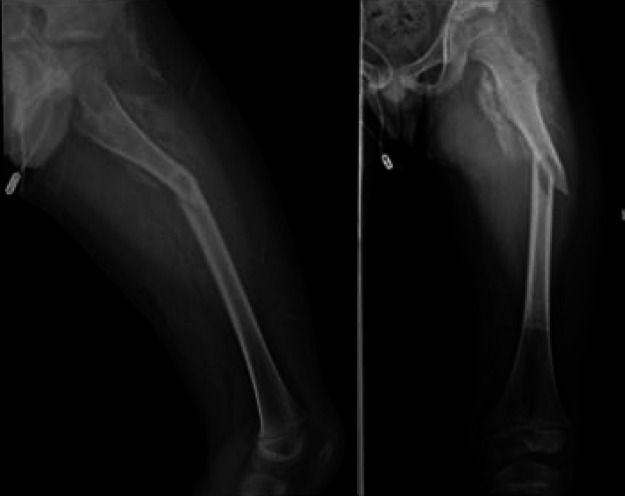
The x-ray of community hospital showed a fracture of the left proximal femur and a massive osteogenesis-like lesion of the left proximal left femur.
